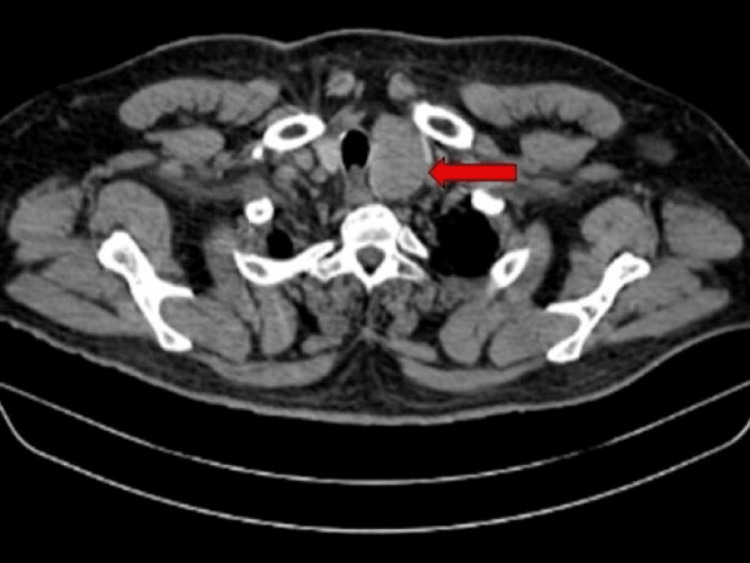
HRCT of the thorax showing a well-defined lesion of size 4.5 x 3.4 x 3.1 cm in the left lobe of the thyroid (red arrow)
